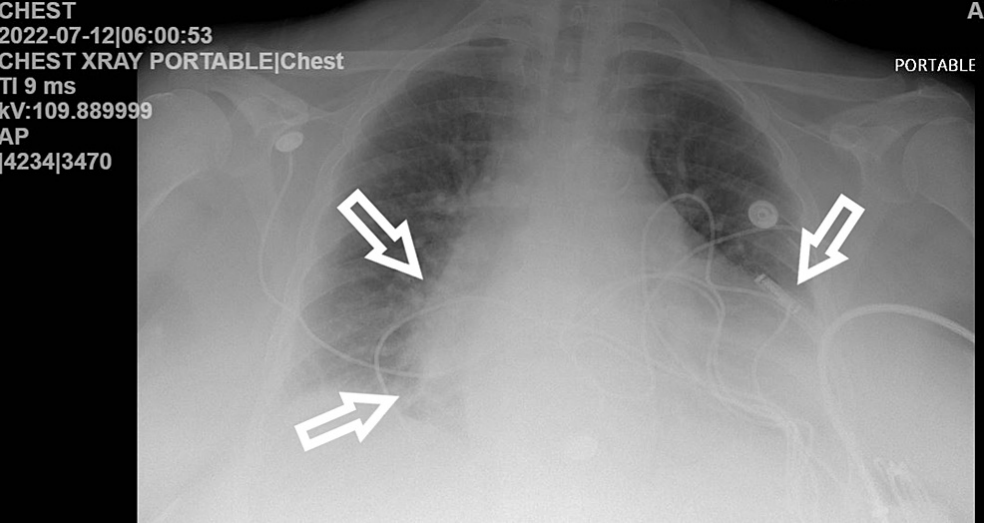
Chest X-ray: consistent with cardiomegaly with hazy perihilar and lower lobe opacities.
